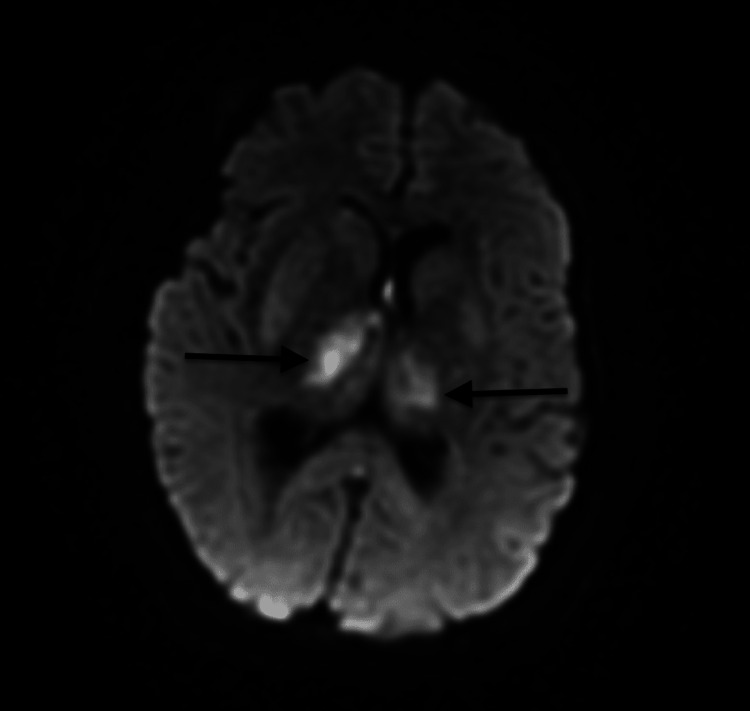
MRI brain (without contrast) MRI brain (without contrast) revealed secondary venous infarcts of bilateral thalami and right basal ganglia. The arrows show bilateral thalami and right basal ganglia venous infarcts.
